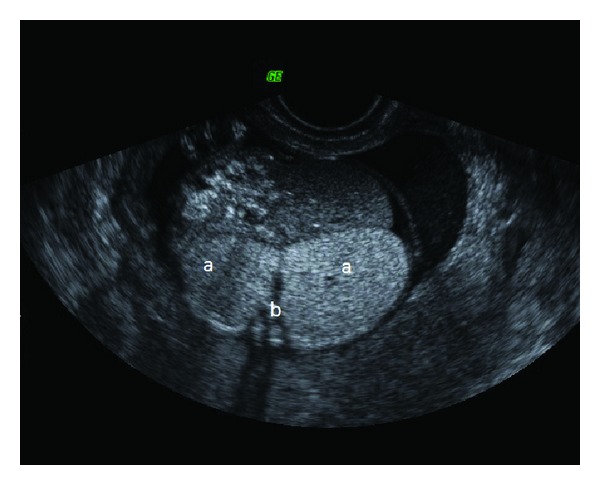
Antenatal sonography of case 2 showing large hyperechoic lungs (a), dilated main bronchi (b), large hyperechoic lungs, inverted diaphragm, and ascites.
